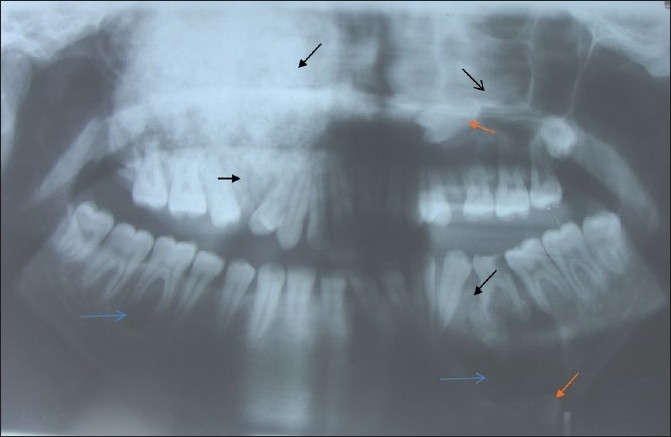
Orthopantomograph: black arrows showing radiopacities, blue arrows showing radioluscent areas and orange arrows showing the impacted premolar
